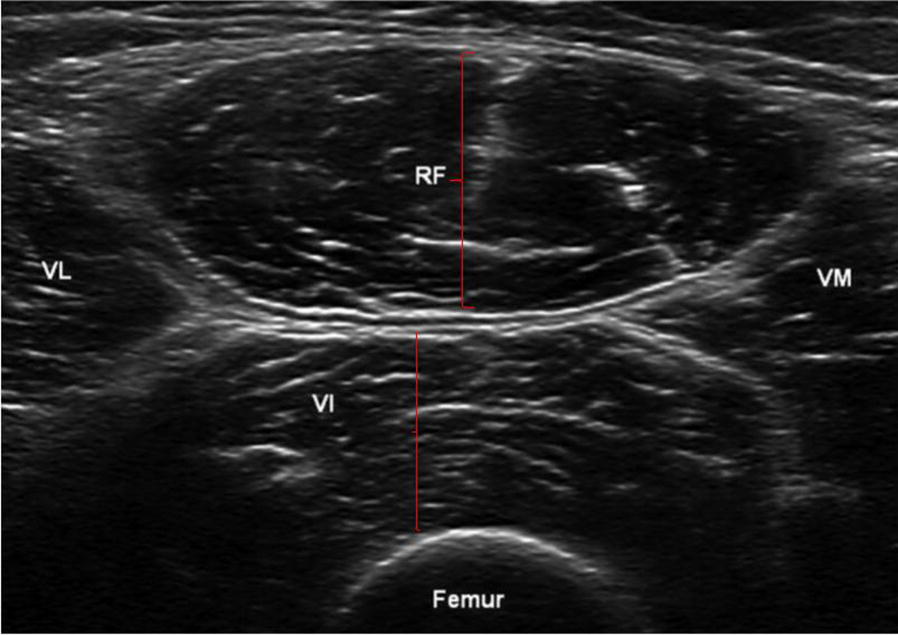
The muscle layer thickness detected by ultrasound. Quadriceps femoris detected by ultrasound in a transverse scan. The rectus femoris (RF) layer thickness and vastus intermedius (VI) are represented (red lines). *VM* vastus medialis, *VL* vastus lateralis
